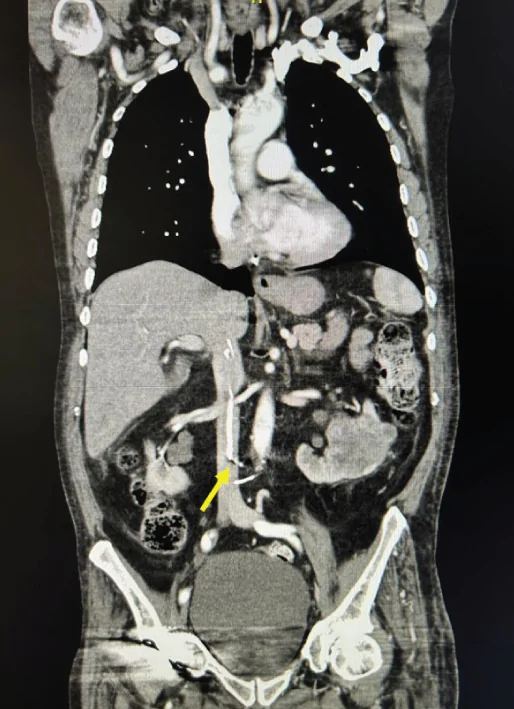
Coronal contrast‐enhanced computed tomography image showing a hyperdense linear structure within the inferior vena cava in continuity with the paravertebral venous plexus, consistent with intravascular cement migration (yellow arrow).
